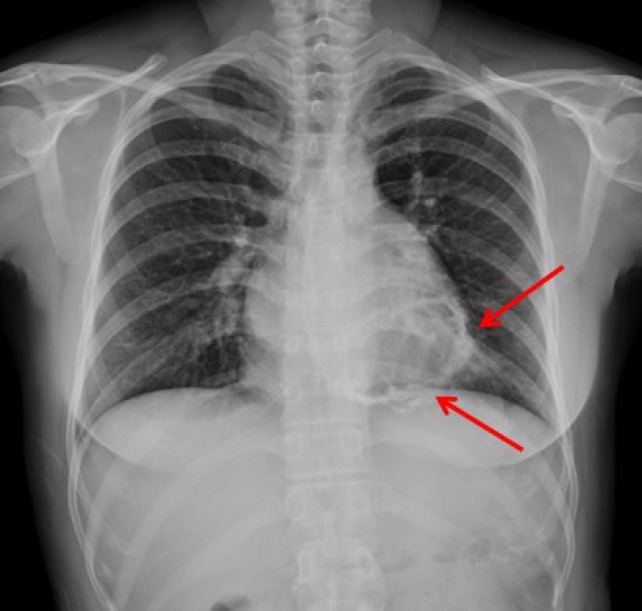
Chest roentgenogram showed normal cardiac size with calcification of the pericardium (arrows).
